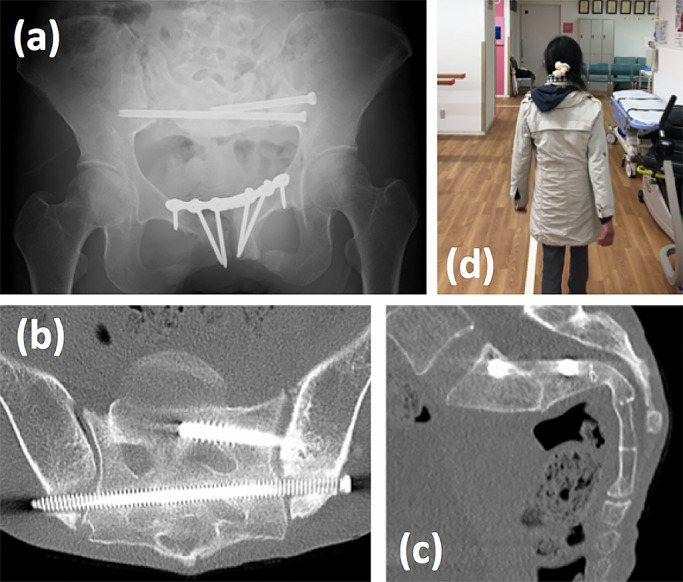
X-ray and CT 6 months after surgery. X-ray of pelvis (a), CT axial plane (b) and sagittal
